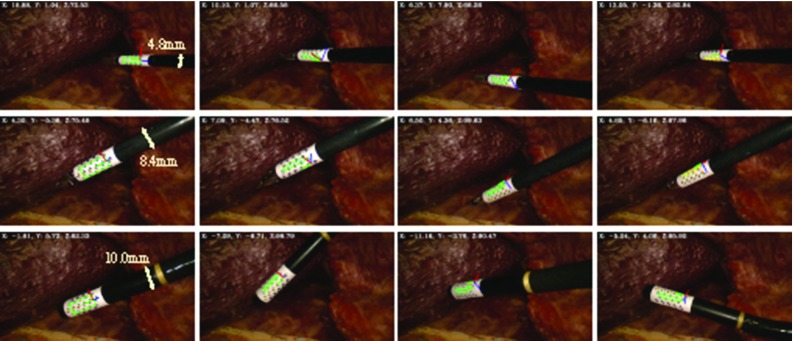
Qualitative results of the tracking framework for the proposed marker on various types of surgical tool. A 5-mm monopolar cautery (*top row*), a da Vinci large needle driver (*middle row*) and a laparoscopic ultrasound probe (*bottom row*) are tracked, respectively. The coordinate axis in *red*, *green* and *blue* shows that the pose has been correctly estimated
